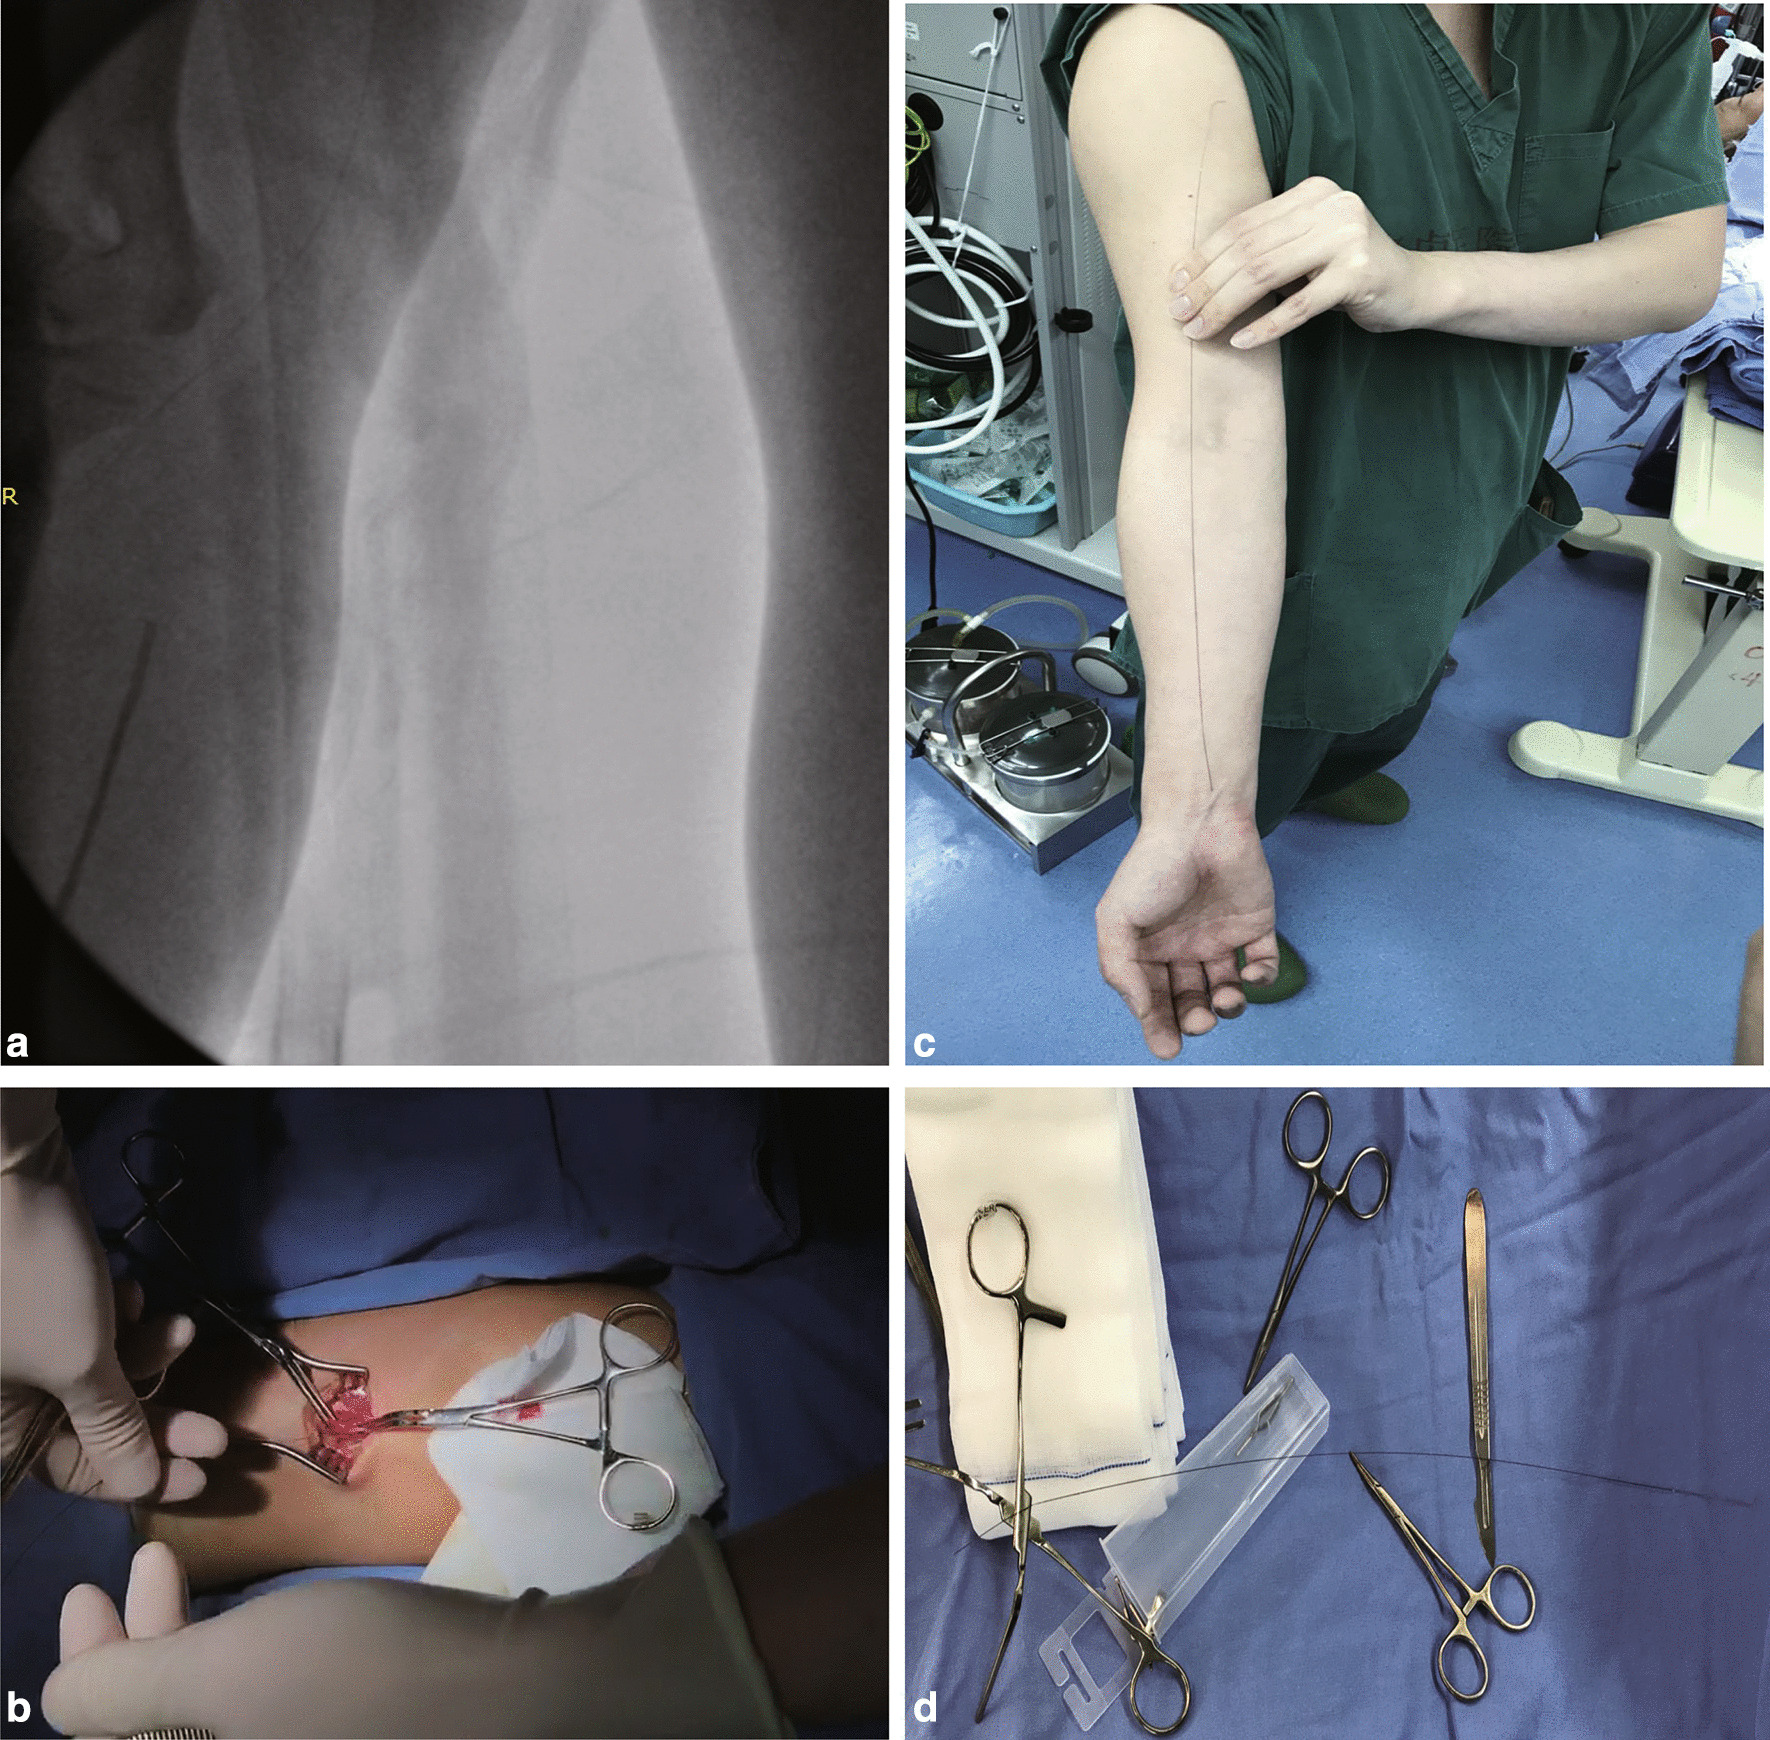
a X-ray showing the broken wire from distal of the radial artery to brachiocephalic artery. b–d The process of retrieve the broken guide from radial artery
